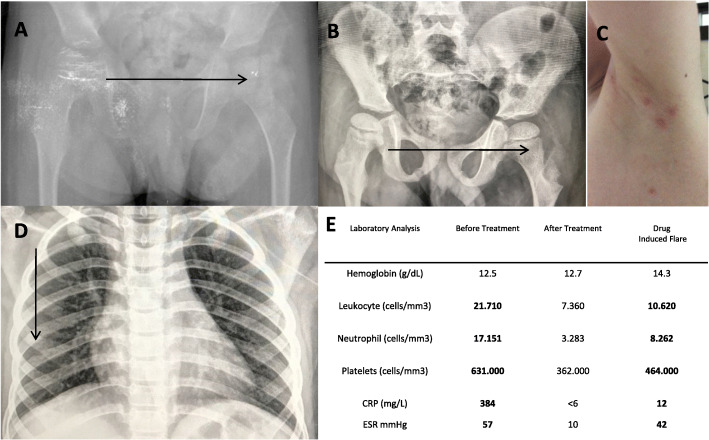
Clinical follow up. Femur x-ray before (a) and after treatment (b) with narrows indicating resolution of severe osteomyelitic lesions and (c) with an initial pustular rash in the forearms observed. The thoracic X-Ray (d) with black narrow indicating an acute osteomyelitis in the 7th rib during a flare induced by canakinumab. The figure (e) shows laboratory control of acute reactant markers before and after the treatment and the inflammation induced by the drug induced flare
